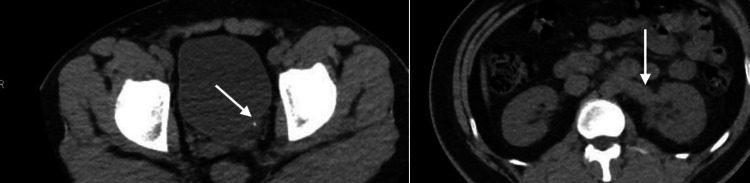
Repeat noncontrast computed tomography performed 10 days later showing the same 4 mm calculus at the left ureterovesical junction (arrow), now with left-sided hydronephrosis and periureteric fat stranding
